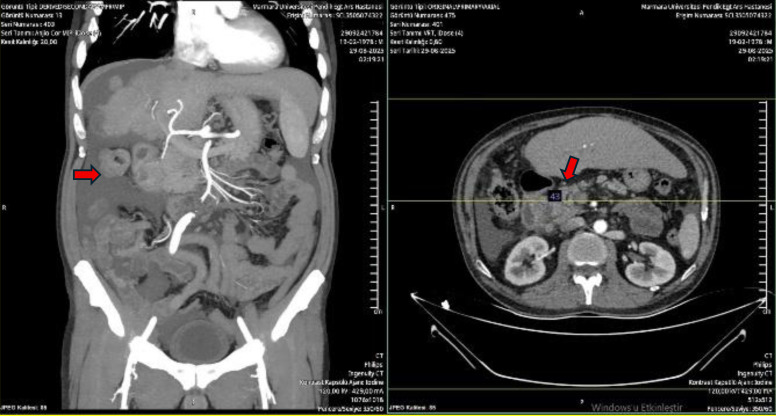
Coronal (left) and axial (right) contrast-enhanced CT images demonstrating a large heterogeneous hyperdense lesion centered in the pancreaticoduodenal groove and extending into the medial wall of the second portion of the duodenum. The lesion is associated with marked luminal narrowing, compatible with intramural duodenal hematoma.
